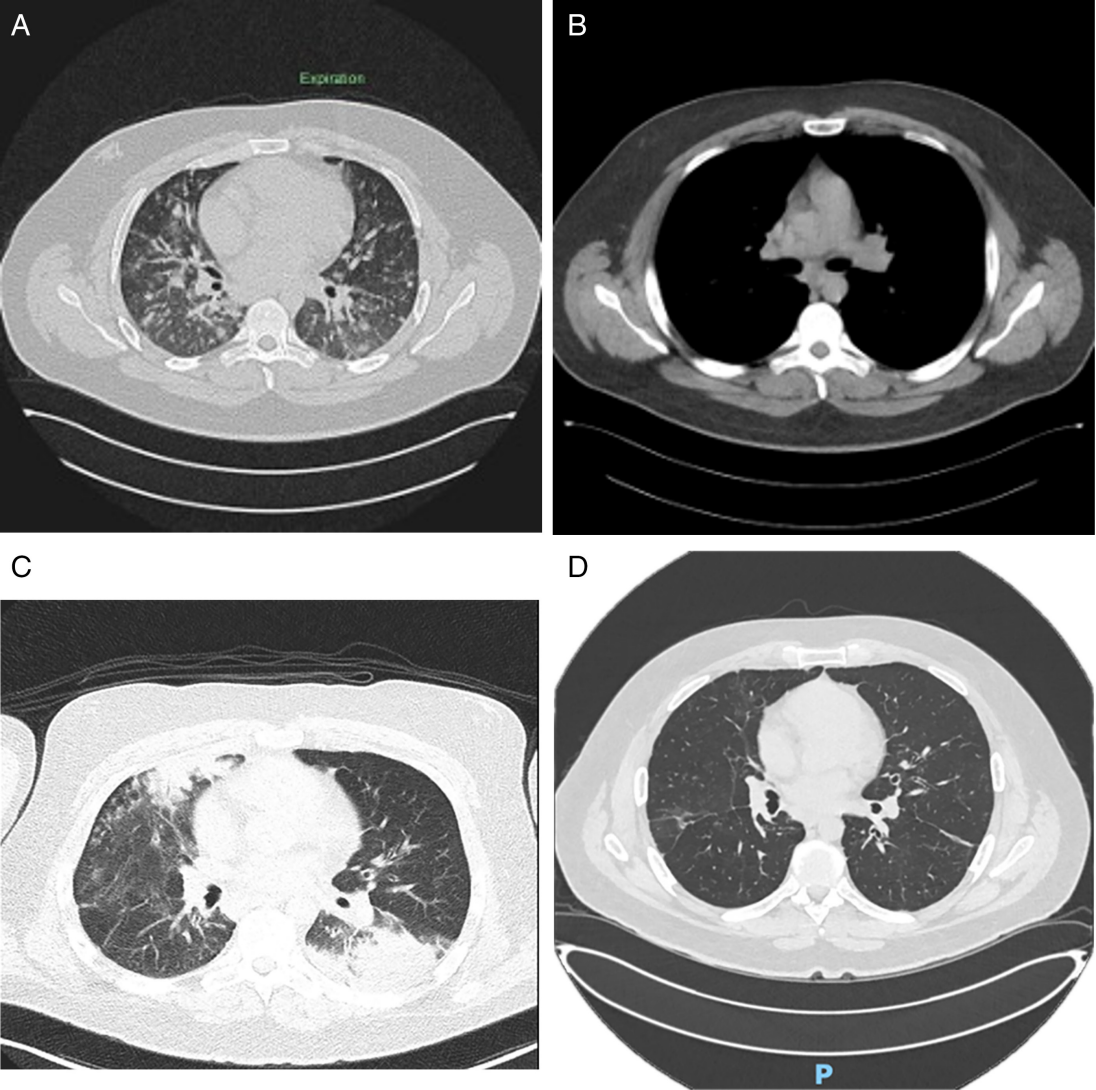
(A) CT chest (November 2017) done at the time of diagnosis demonstrating multiple peribronchovascular, subpleural and perifissural nodular ground‐glass infiltrates within the mid to lower zones. (B) CT chest (November 2017) demonstrating extensive mediastinal lymphadenopathy. (C) CT chest (September 2023) demonstrating dense consolidation left lower lobe and right upper lobe done. (D) CT chest (May 2024) demonstrating resolution of consolidation following intravenous immunoglobulin (IVIG) treatment.
